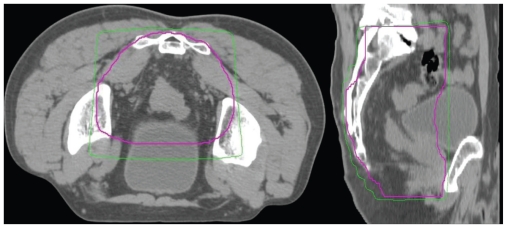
Axial and Sagittal phase I planning CT showing planning target volume (thick line) and 95% isodose (thin line). Note a higher than standard superior border to cover primary disease with a 3 cm margin.
